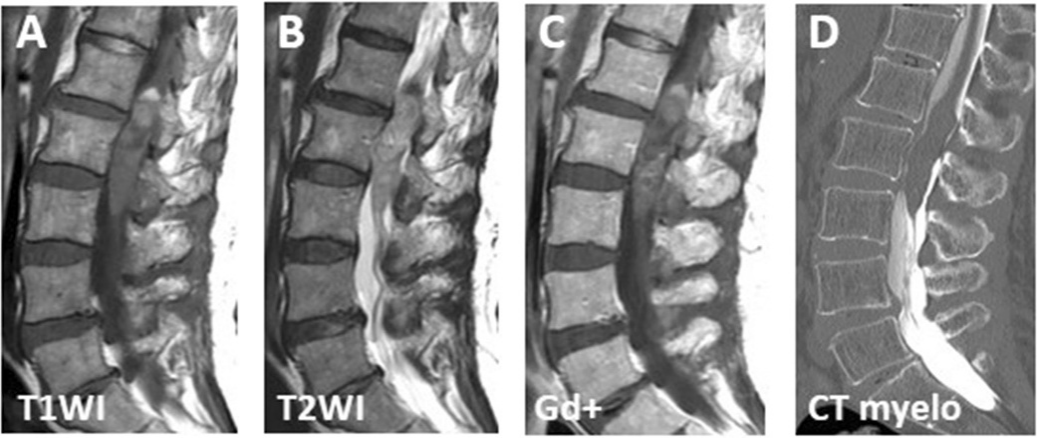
Preoperative MRI scan: preoperative radiological findings. An MRI scan revealed an intramedullary mass lesion extending from vertebral level L1 to L3 with mixed iso- and high-intensity change in T1-weighted image (A) and with isointensity change in the T2-weighted image (B). Upon gadolinium enhancement, the mass lesion was heterogeneously enhanced (C). A CT myelography showed a filling defect at the L1 to L3 vertebral levels (D).
